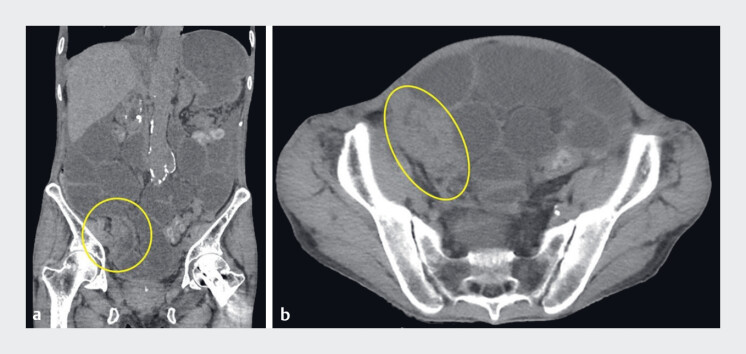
Computed tomography findings before stent placement showed thickened walls in the ileocecal region (indicated by the yellow circle), with proximal bowel dilation.
